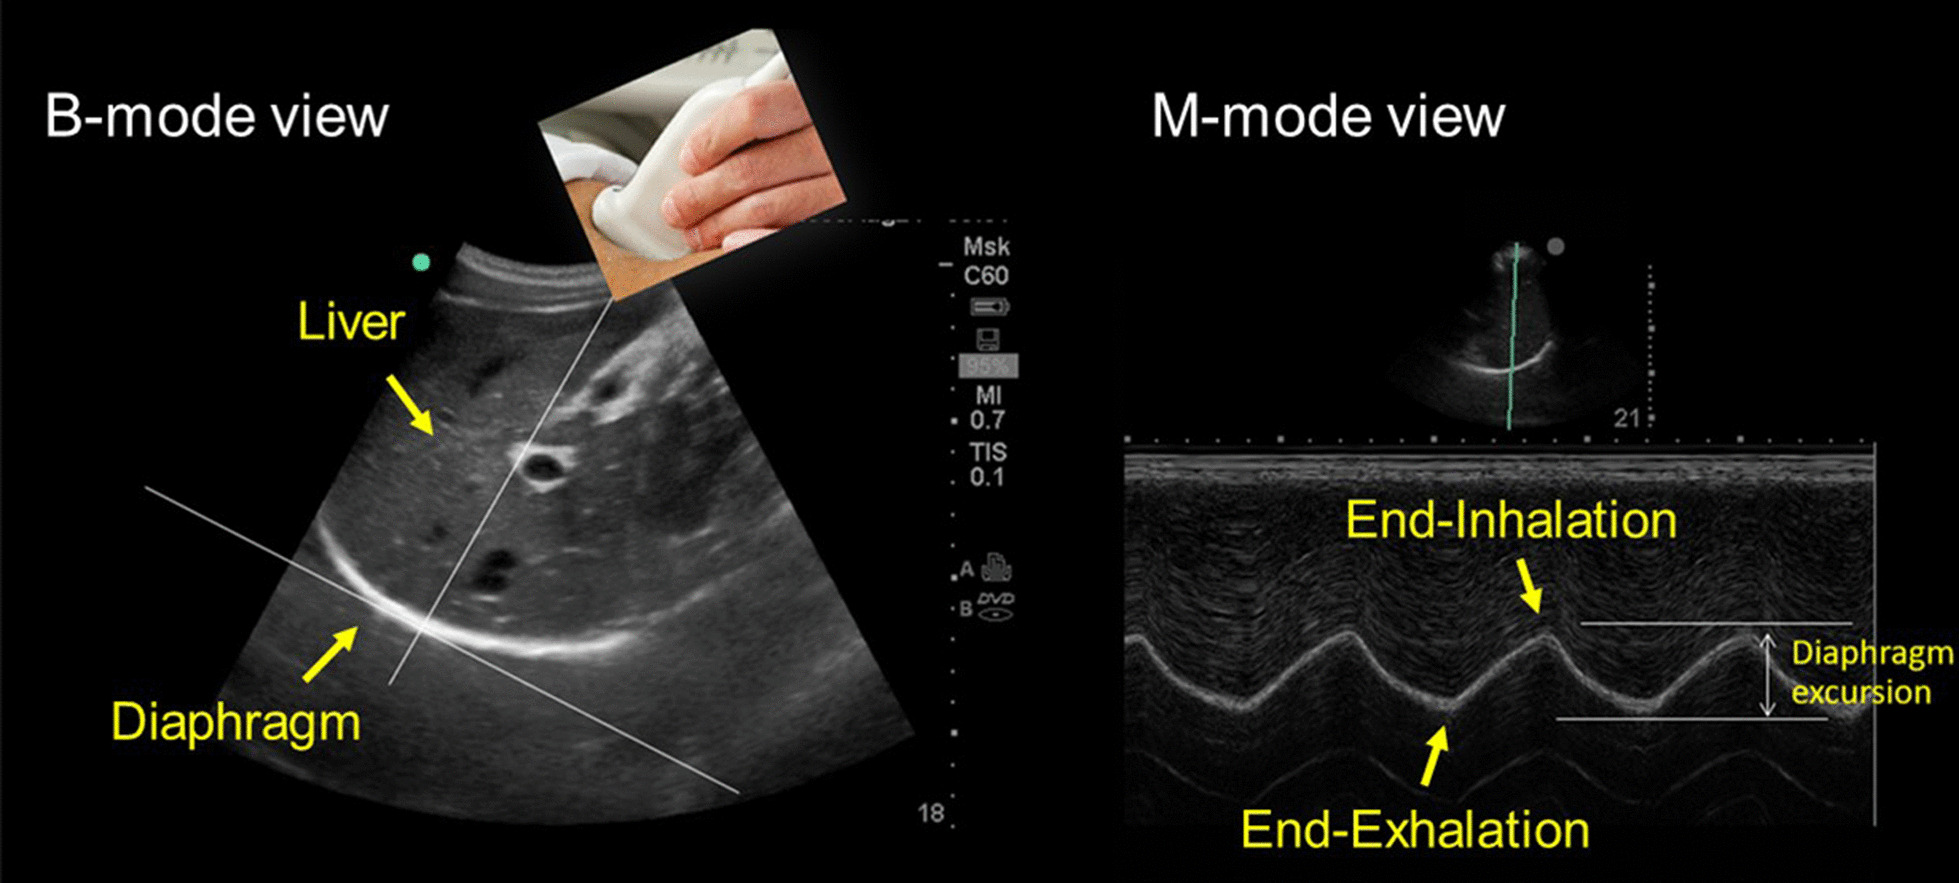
Ultrasound image of the dome of the diaphragm in brightness-mode (B-mode; *left panel*) and motion-mode (M-mode; *right panel*). As the diaphragm contracts, the dome moves towards the ultrasound probe. The larger the caudal displacement of the diaphragm, the greater the diaphragmatic contribution to tidal breathing. Reproduced with permission from The American Association for Respiratory Care: Shaikh e al. Respir Care 2019;64:1600–2. The entirety of both images was obtained by Dr. Shaikh
